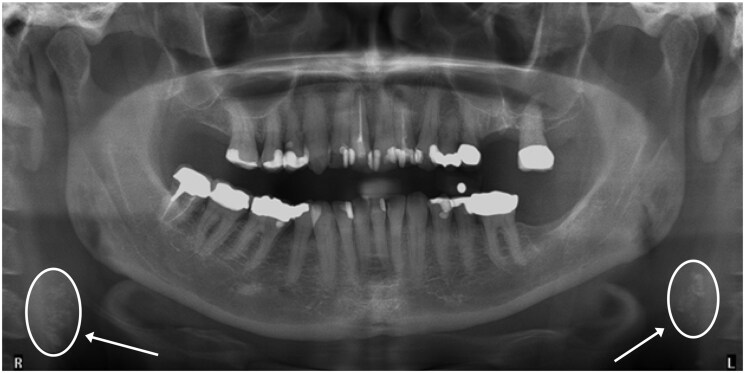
Bilateral calcified carotid artery atheroma (enhanced and marked with white rings) depicted on a panoramic radiograph.
